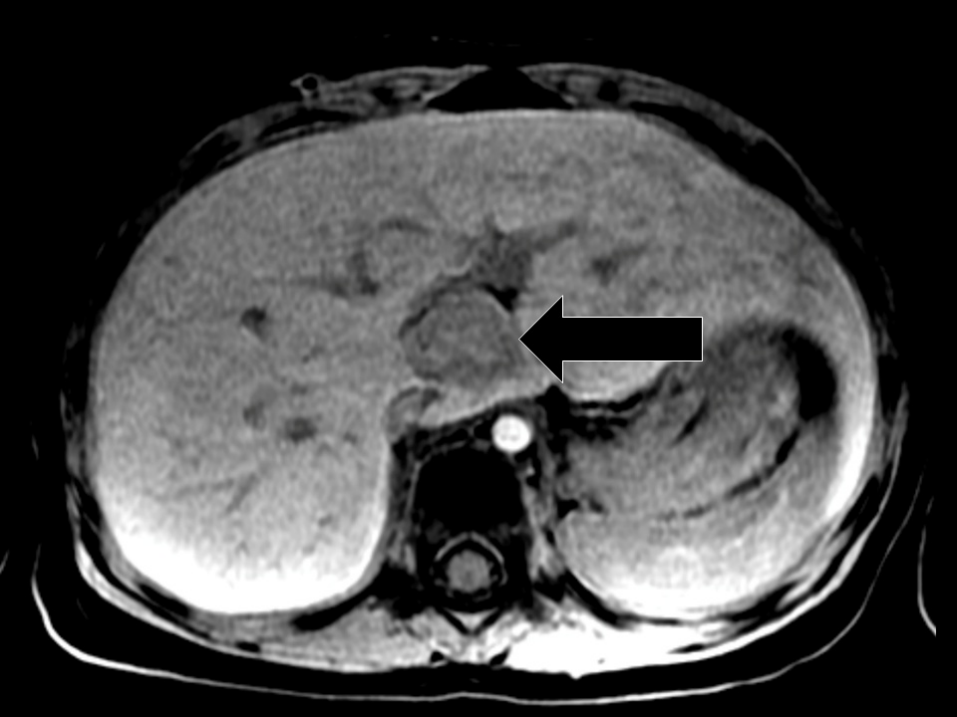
Axial T1-weighted Sequence Obtained after Intravenous Injection of Eovist Axial T1-weighted sequence obtained 20 minutes after intravenous injection of the hepatobiliary imaging agent Eovist (gadoxetate disodium). There was variable Eovist uptake in the liver lesions seen on T2-weighted imaging. In this centrally-located lesion (indicated by arrow), there is poor Eovist uptake compared with background liver parenchyma. Functional hepatocytes are required for Eovist uptake, and FNH typically contains functional hepatocytes; therefore, poor Eovist uptake is concerning for a non-hepatocyte-containing neoplasm. In this patient, this imaging expectation led to a false positive result. FNH - focal nodular hyperplasia.
